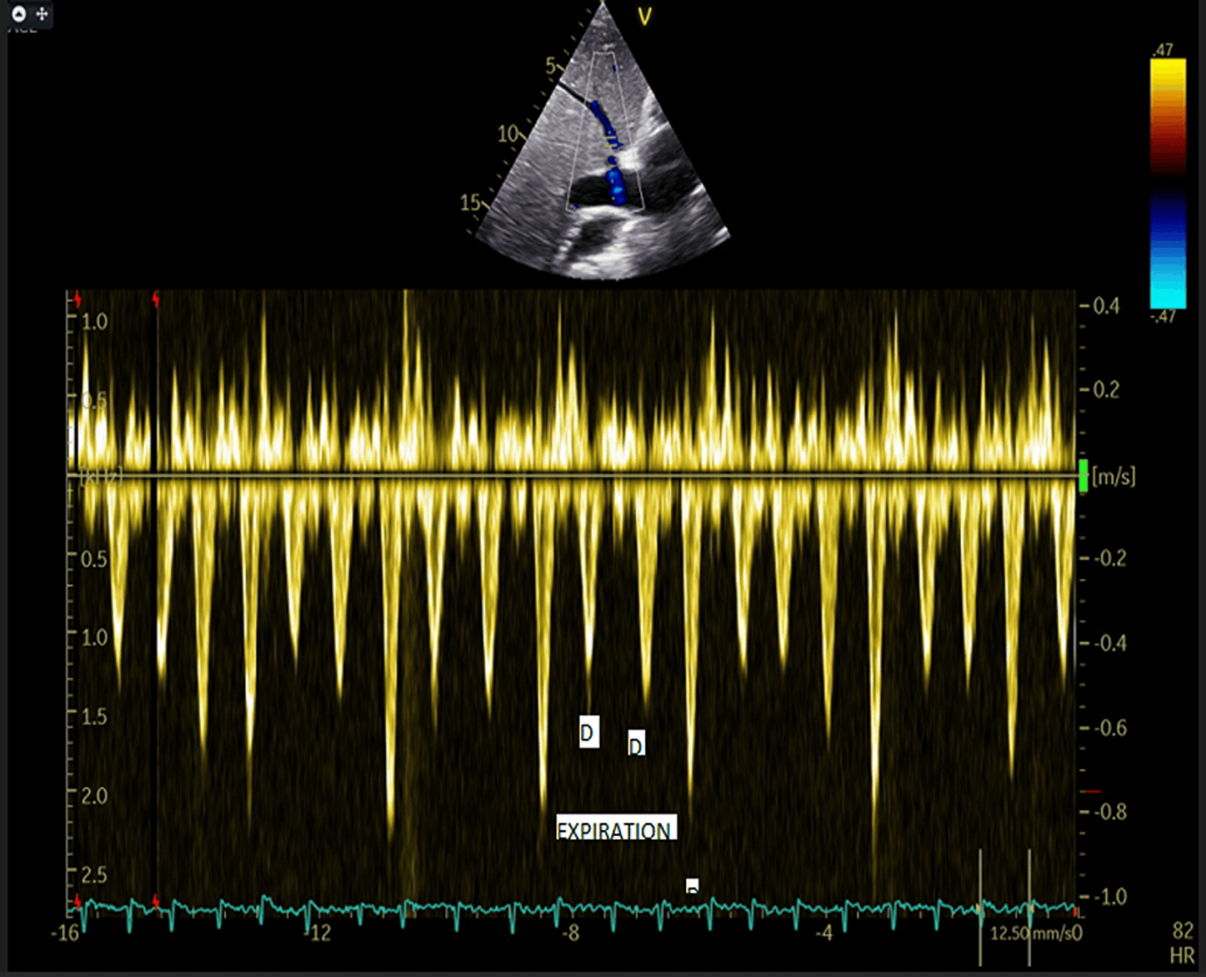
Echocardiography The subcostal view at the level of the hepatic veins shows respiratory variations
